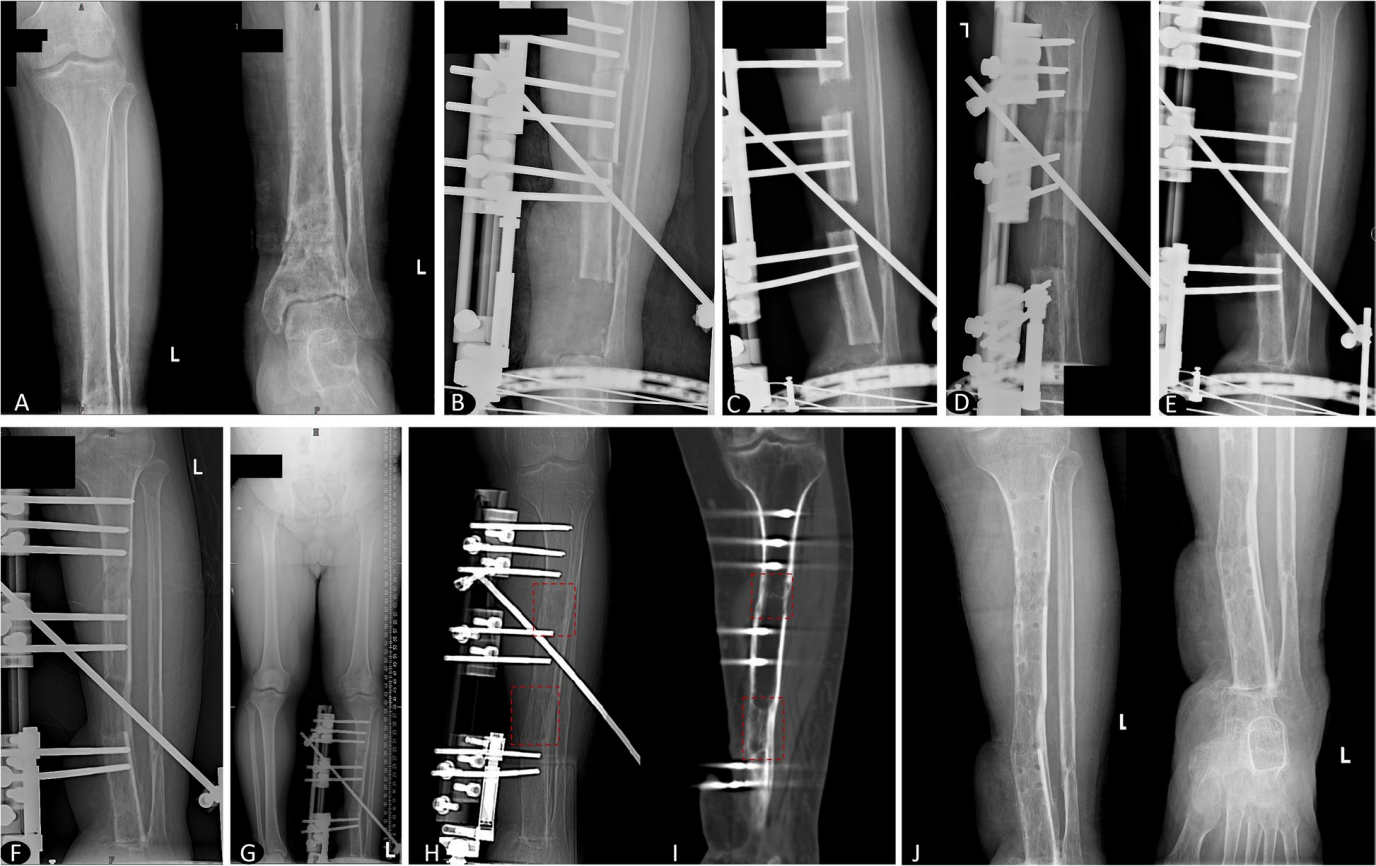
A A 28-year-old male patient with postoperative infection in open tibial fractures. B An excision of infected bone and soft tissue with 7.3 cm defect and beginning of trifocal bone transport. C One month after trifocal bone transport. D, E, F 1 month, 3 months, 6 months after the docking was reached. G, H, I Trifocal bone transport was completed with good regenerate consolidation, and docking union was achieved and evaluated on X-ray and CT. J Radiograph appearance with an excellent bone result at 2 years after the removal of the external fixator
